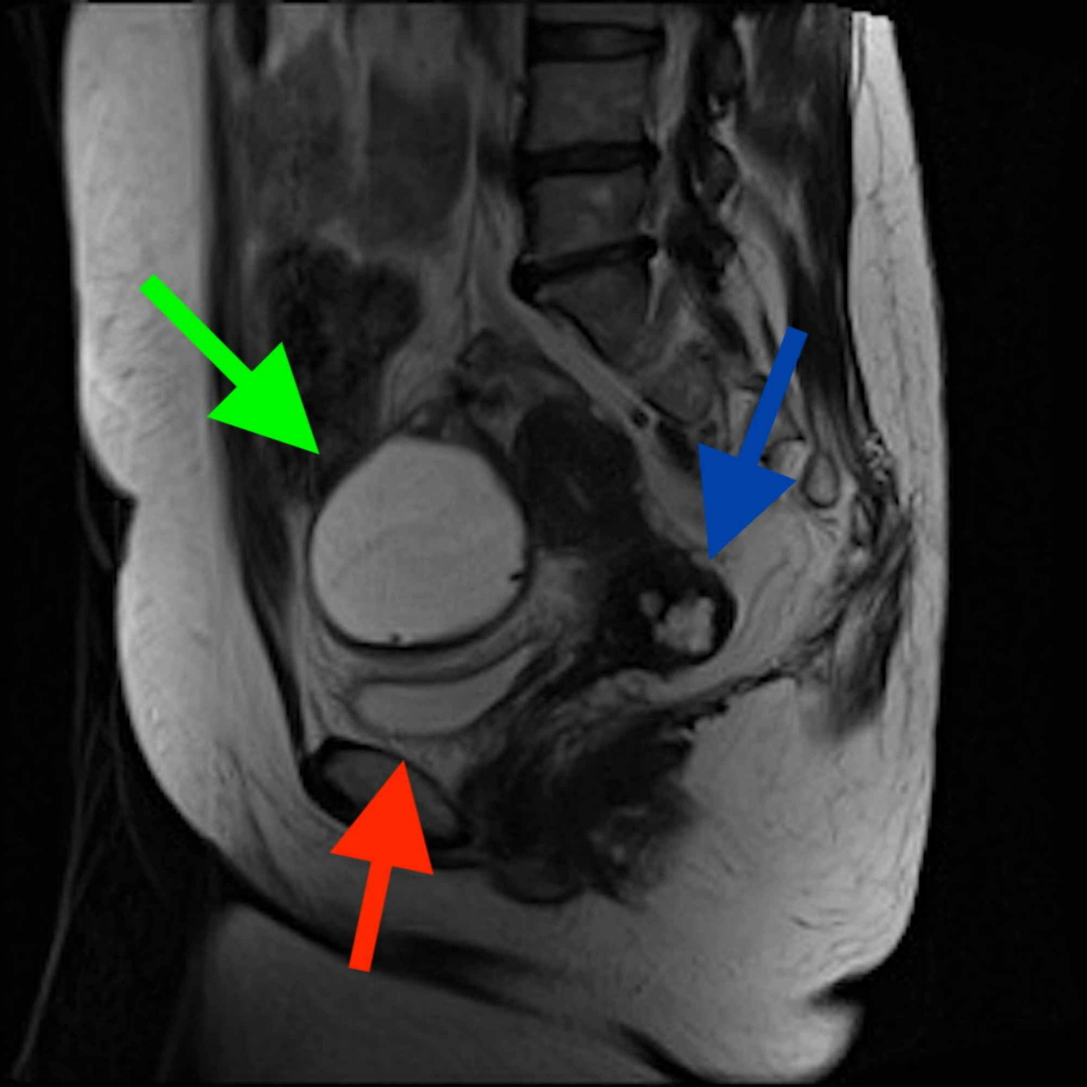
MRI sagittal plane view of ovarian cyst (green arrow) causing mass effect on bladder (red arrow). Nabothian cysts (blue arrow) also noted.
